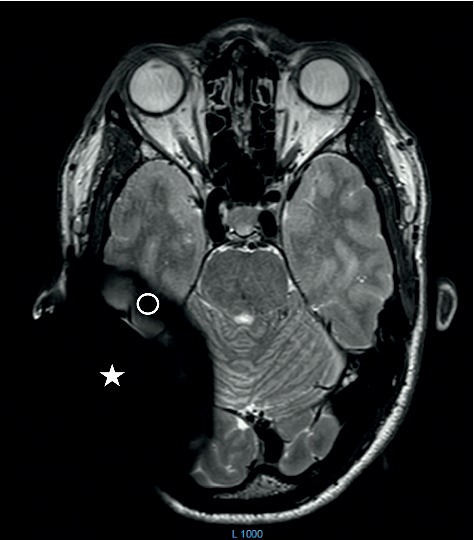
MRI survey pattern of the sagittal plain of a 1.5 T scanning of an Advanced Bionics 3D Implant.
